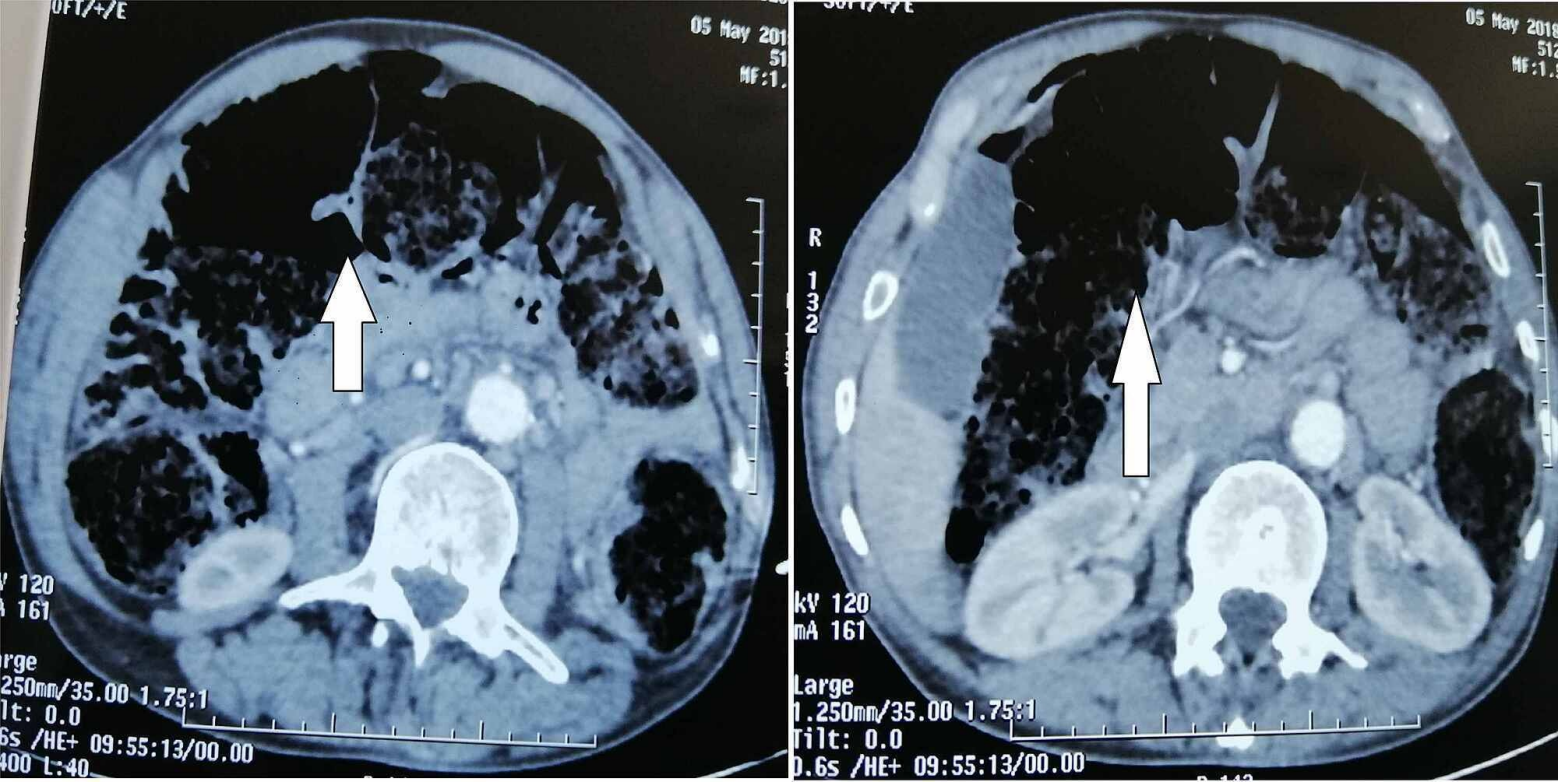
Abdominal CT scan Axial CT scan showing a distention of small and large bowel (White arrow).
